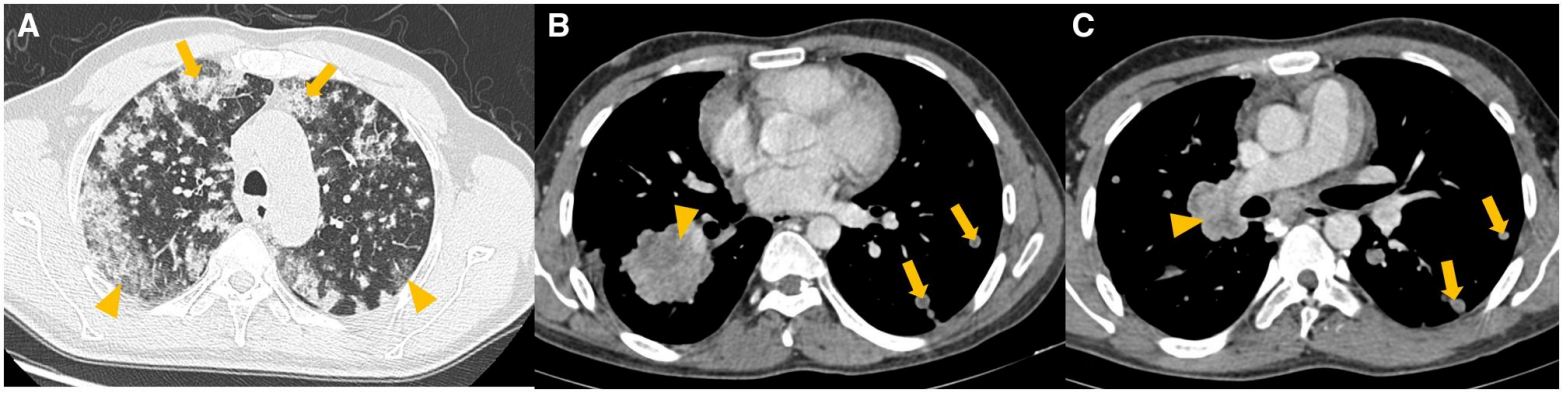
(A-C) Contrast enhanced computed tomography (CECT) lung with epidermal growth factor receptor (EGFR) and anaplastic lymphoma kinase (ALK) mutation positive lung adenocarcinomas. (A) CECT thorax shows multifocal discrete and confluent nodules; some pure ground glass (arrowheads) and some part-solid (arrows), in this 60 years old female (without any history of smoking) with biopsy proven EGFR mutation positive lung adenocarcinoma. (B, C) CECT thorax (mediastinal window) of a 50 years old male (without any history of smoking) with biopsy proven ALK mutation positive lung adenocarcinoma, shows a solid right lower lobe mass with irregular margins (arrowhead in B) and metastatic left lung nodules (arrows in B, C). In addition, metastatic right hilar nodal mass (10R station) is seen (arrowhead in C).
